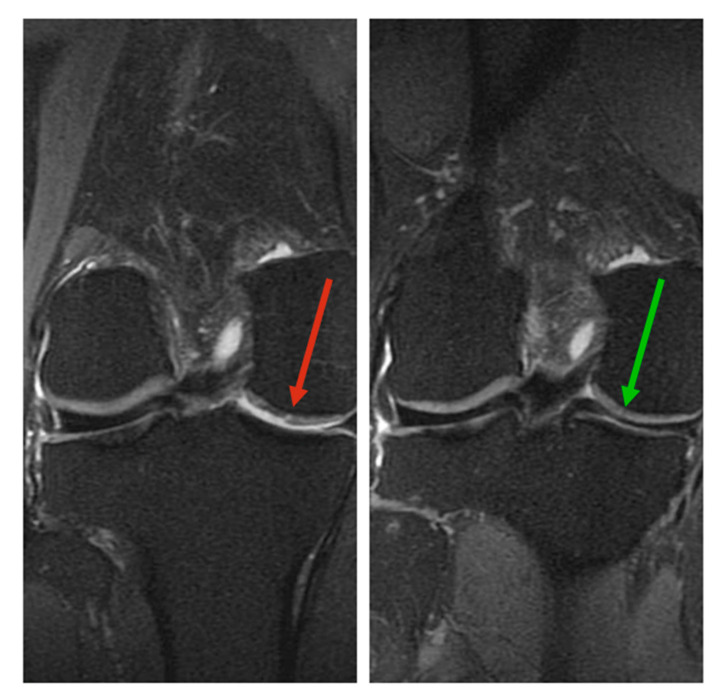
Magnetic resonance imaging (MRI) status of the right knee joint before treatment with the SVF (left), showing lesion in the cartilage (red arrow) and 16 months after treatment with SVF (right), showing potential cartilage regeneration and disappearance of the effusion (green arrow).
